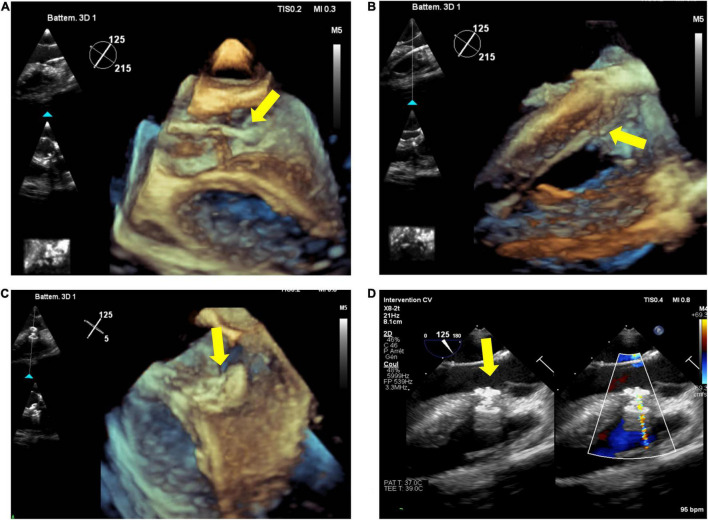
Percutaneous closure of perimembranous ventricular septal defect (pmVSD). Transesophageal echocardiography (TEE), especially 3D, permits visualization of catheter passage through the aortic valve (A) and in the right ventricle (B) (yellow arrow), and live monitoring during (C) and after device release (D) (yellow arrow).
